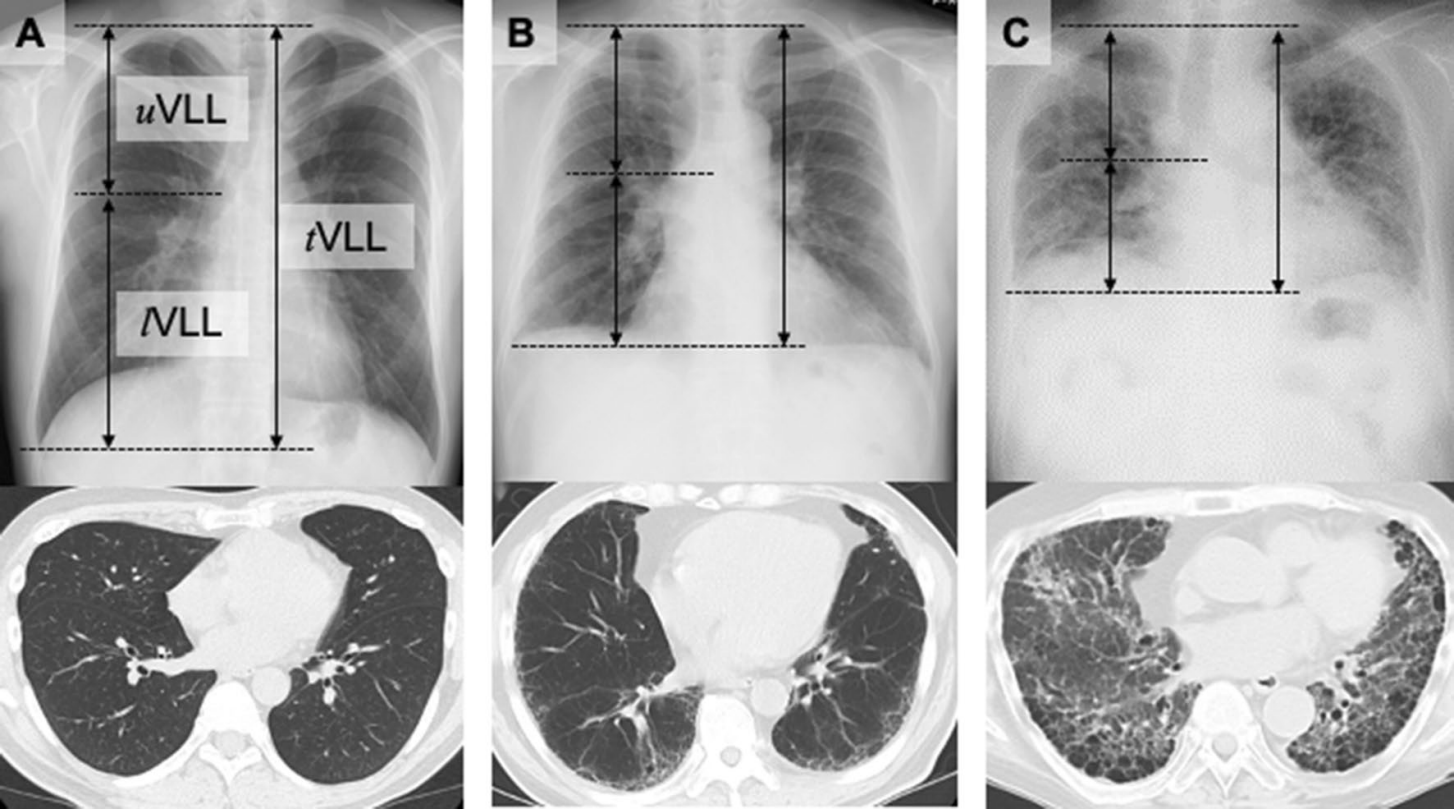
Measurements of vertical lung length (VLL) on chest X-rays. Three VLL measurements were taken: upper VLL (*u*VLL), from the apex to the costophrenic angle; lower VLL (*l*VLL), from the tracheal carina to the costophrenic angle; and total VLL (*t*VLL), from the apex to the costophrenic angle. Measurements were performed in the right lung. Compared with control subjects (A), patients with mild interstitial pneumonia (IIP) (B) and severe IIP (C) demonstrated decreased *u*VLL, *l*VLL, and *t*VLL. The decrease in *l*VLL was especially notable.
